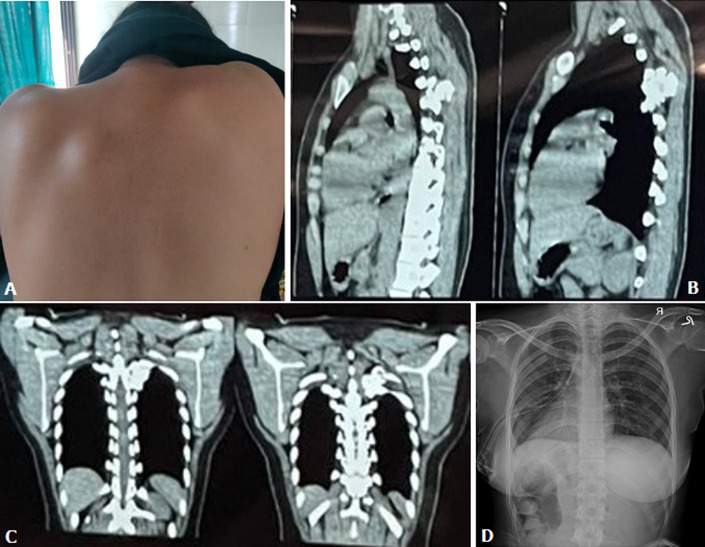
A) clinical picture of the swelling in the left 4^th^ intercostal region; B) sagittal section of CT thorax showing the irregular mass between the 4^th^ and 5^th^ rib; C) coronal section of CT thorax showing the irregular mass between the 4^th^ and 5^th^ rib; D) post-operative X-ray with resected mass and the costovertebral junction of the 5^th^ rib
